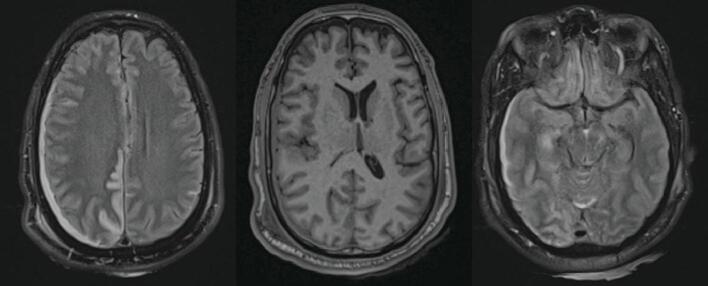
MRI Head on Hospital Day 2 T2-weighted MRI Head on HD2 showing multifocal acute infarcts secondary to herniation, 6 mm midline shift and stable transtentorial herniation.
